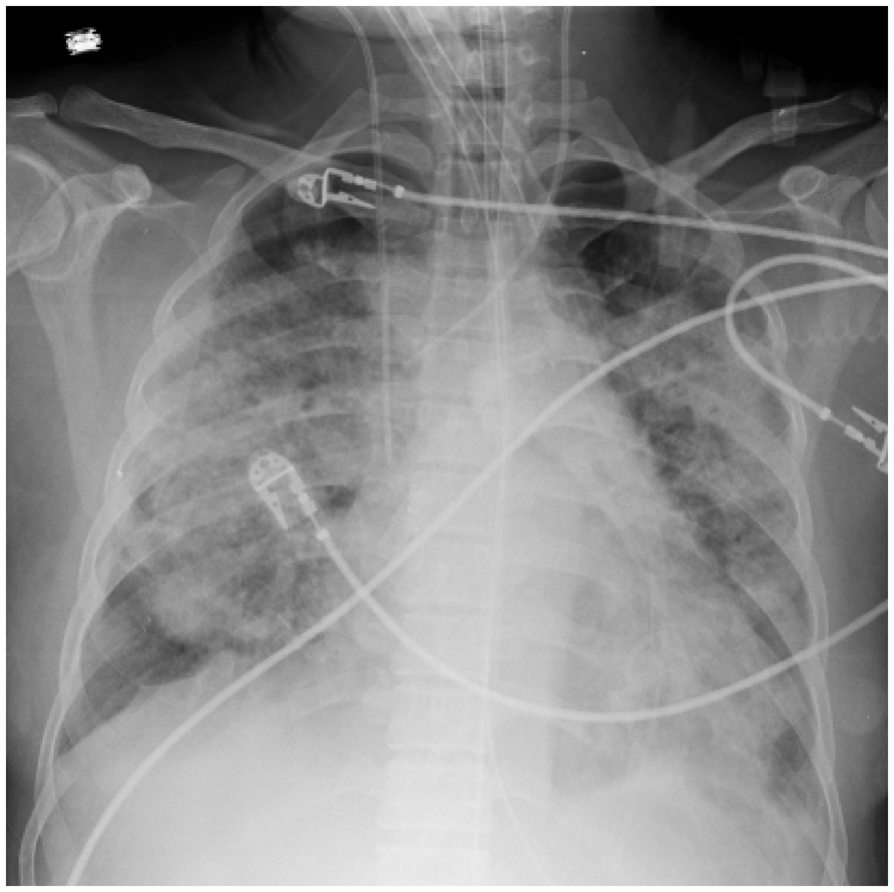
Chest x-ray representing acute lung injury.
